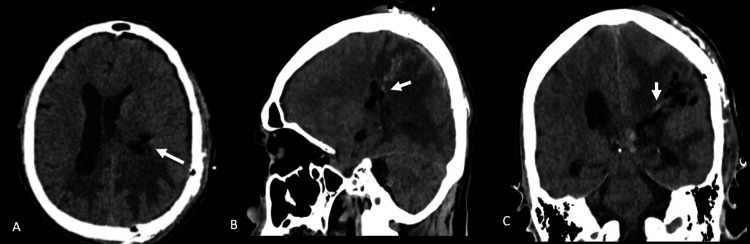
Immediate postoperative CT scan (A) Axial, (B) sagittal, and (C) coronal CT images showing small intraventricular air and minimal blood products within the left lateral ventricle atrium (white arrows), without parenchymal hemorrhage or ventricular dilation. No postoperative hydrocephalus is observed.
